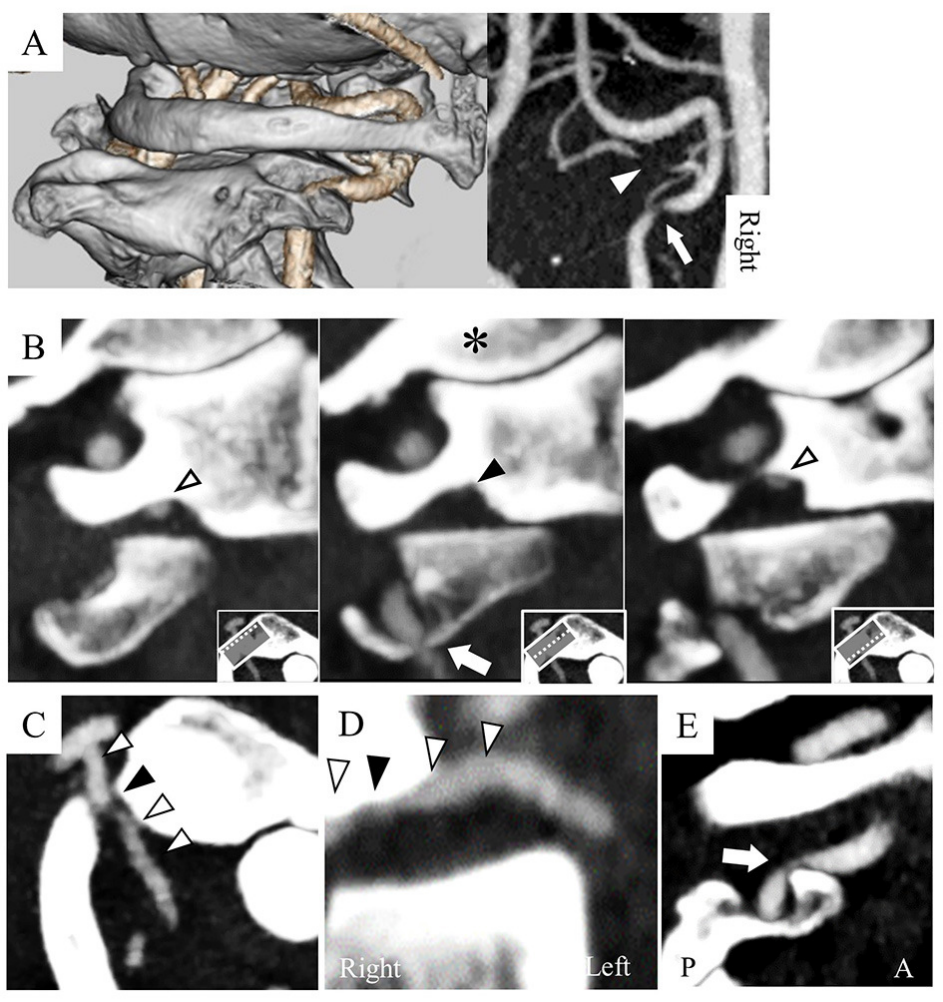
Computed tomography angiography (CTA) with leftward head rotation shows the right PICA and VA as compressed (A). The PICA was compressed in the narrow space surrounded by the posterior arch and lateral mass of the atlas and the superior articular facet of the axis (B: sagittal image of the atlas; C: axial; D: coronal). The right VA was compressed by the anterior wall of the transverse foramen at the axis (E: sagittal image of the axis). White arrowhead: PICA, black arrowhead: compressed PICA, arrow: compressed VA, *: occipital condyle, A: anterior, P: posterior.
